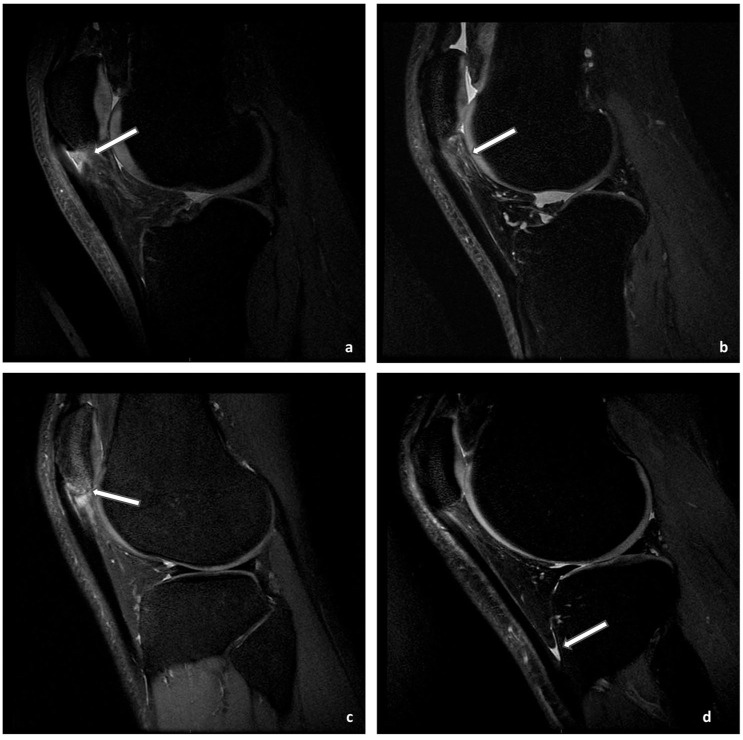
Sagittal, fat-suppressed proton density MRI scans: (a) focal tendon disruption (arrow), (b) grade 2 IFP (or Hoffa fat pad) edema (arrow), (c) the presence of bone marrow edema (arrow), and (d) the presence of deep infrapatellar bursitis (arrow). These images were from different athletes with PT. IFP, infrapatellar fat pad; MRI, magnetic resonance imaging; PT, patellar tendinopathy; US, ultrasound.
